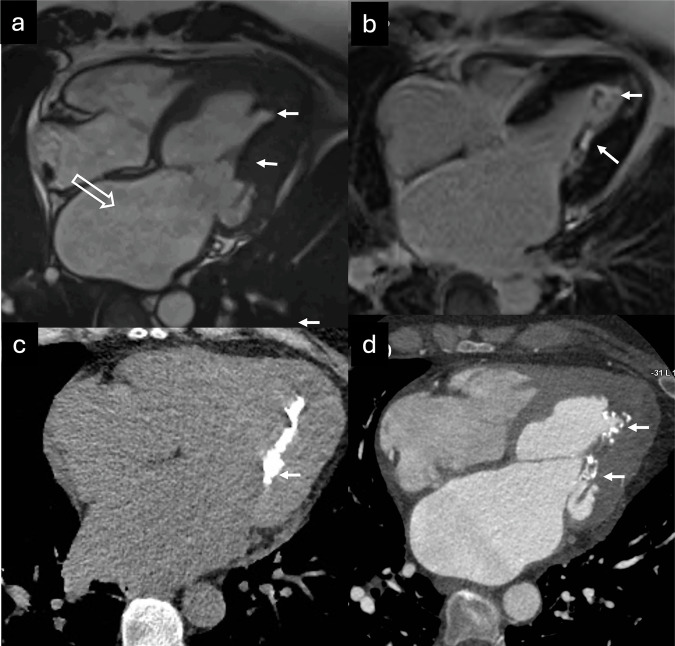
A 52-year-old patient with chronic EMF. CMR (a SSFP cine four-chamber view and b LGE image) and cardiac CT (c noncontrast and d contrast-enhanced images) show partial obliteration of the LV cavity by fibrosis extending to the papillary muscles and an intracavitary thrombus (solid arrows), which may mimic ETs. Note the associated left atrial dilation (hollow arrow in a) and partial calcification of the intracavitary thrombus and fibrotic myocardium (arrows in c, d)
